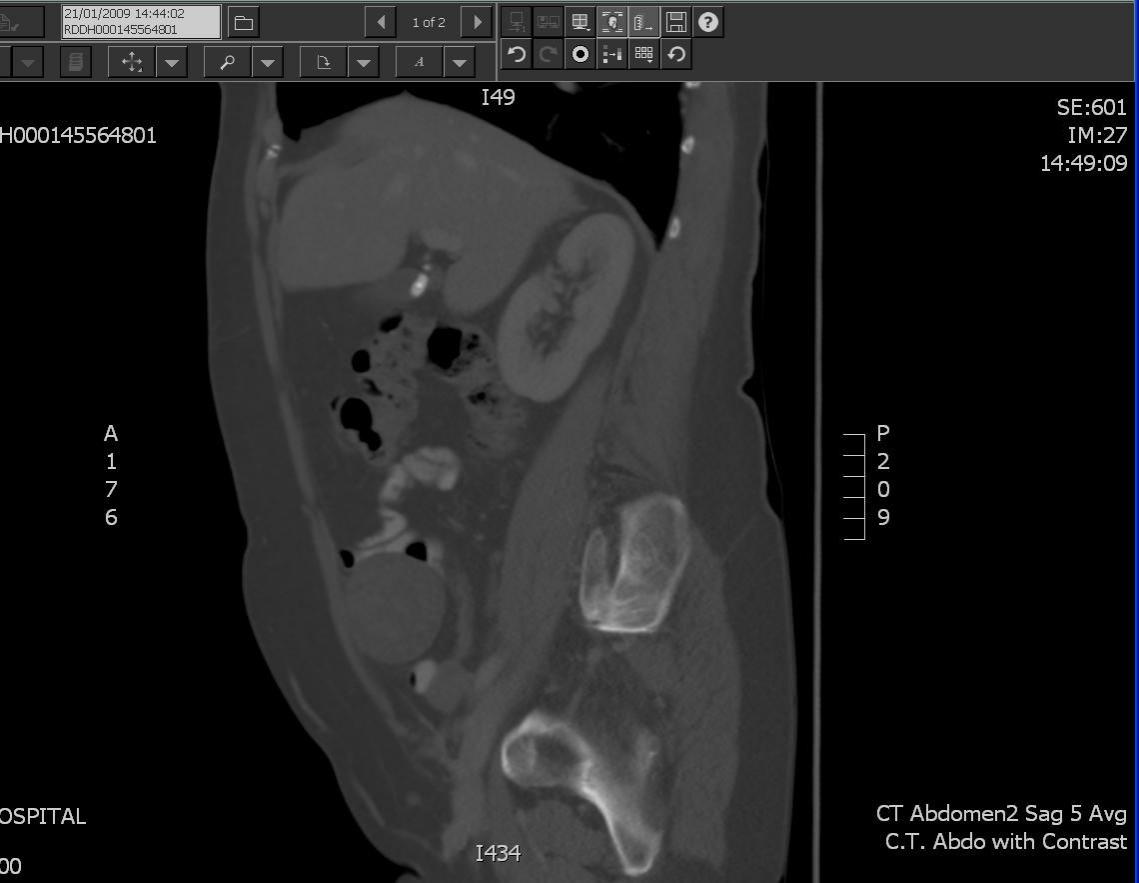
Saggital section of CT Abdomen with Contrast. Well circumscribed RIF mass next to anterior abdominal wall.
